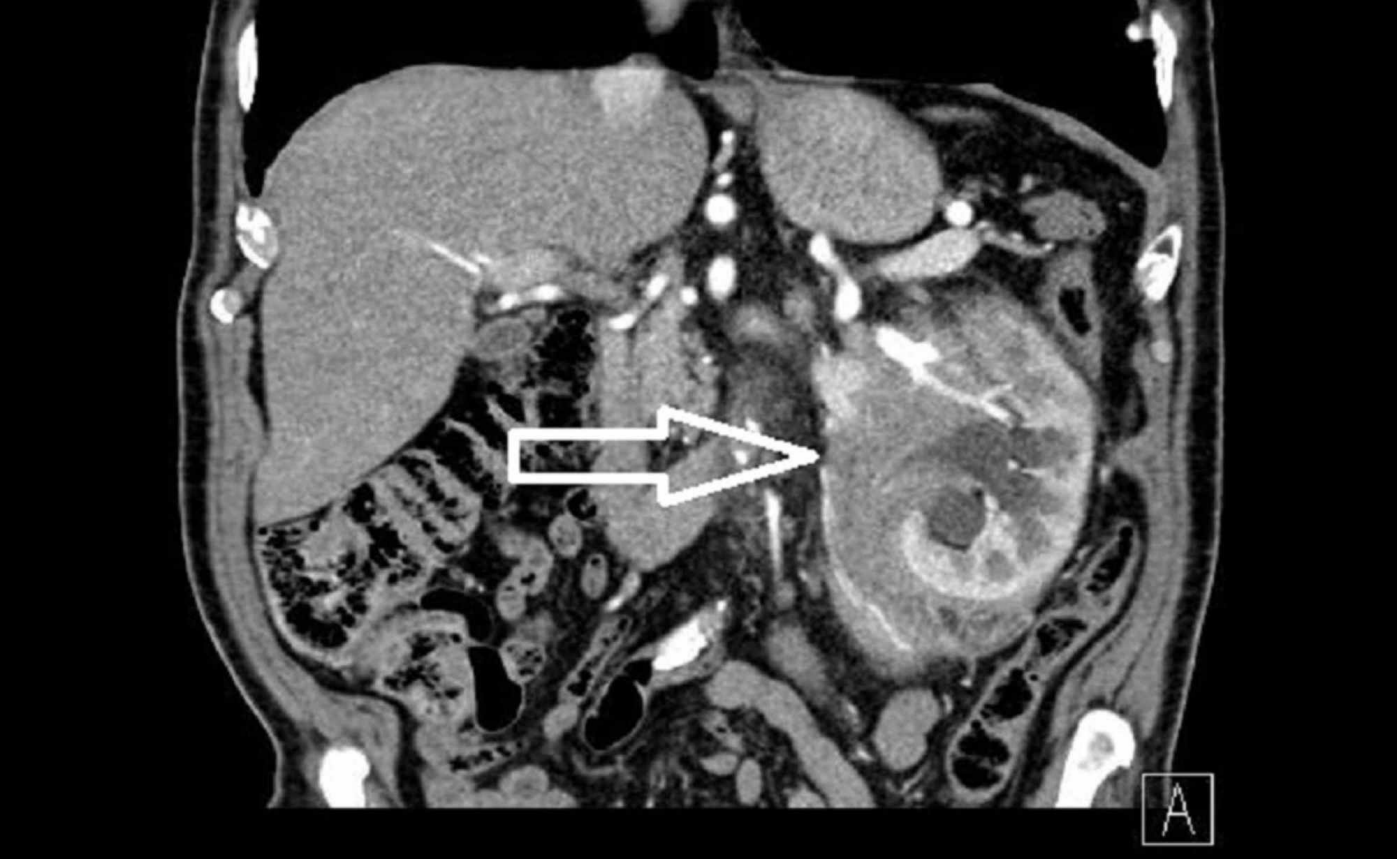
Computed tomography finding (arrow)
